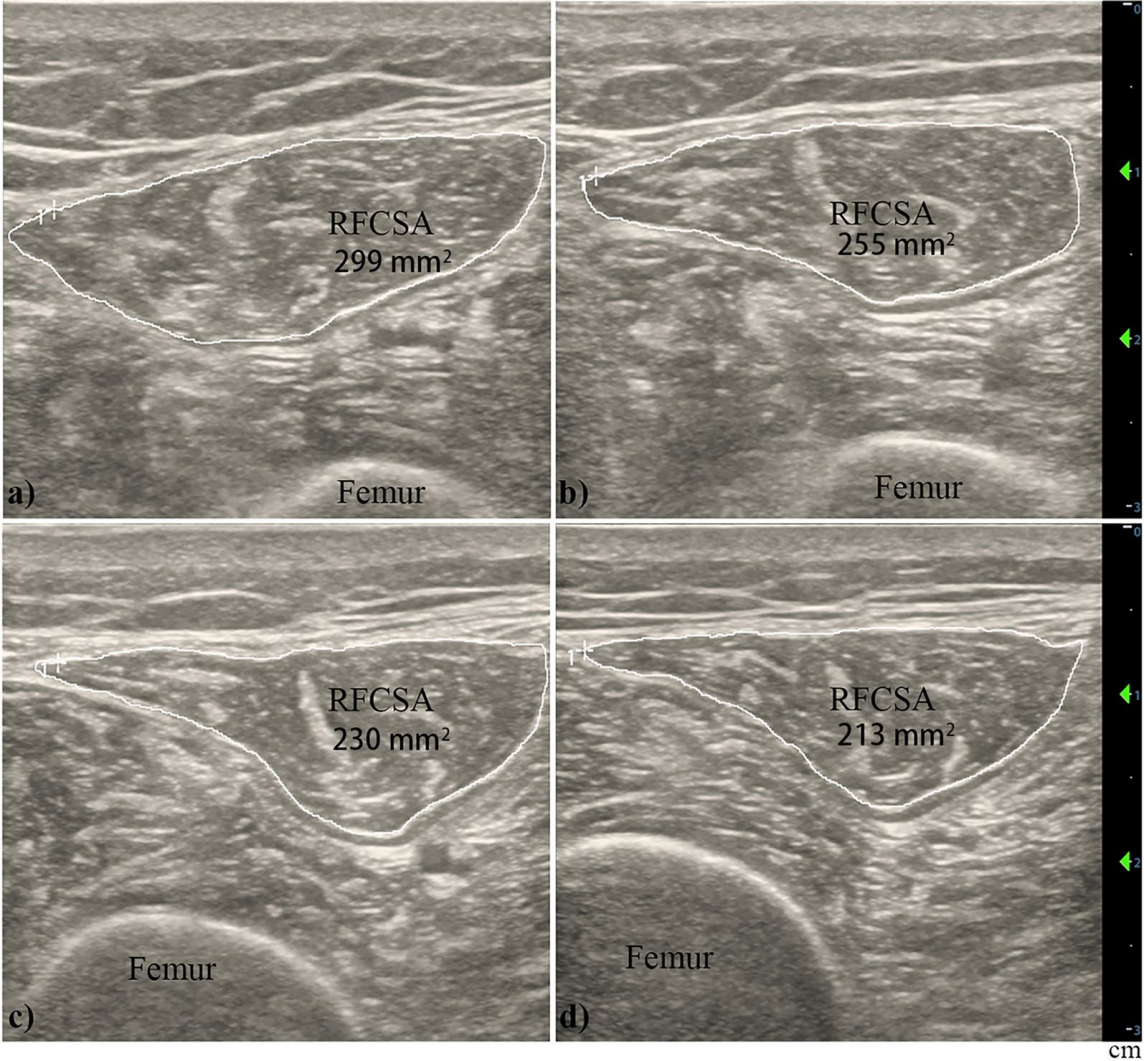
Ultrasound measurements of RFCSA among patients undergoing invasive mechanical ventilation. The RFCSA is outlined by a white border in each image. RFCSA on D1 of ICU admission (a), RFCSA on D3 (b), RFCSA on D5 (c), and RFCSA on D7 (d). The gain and depth settings were consistent across all images. RFCSA, rectus femoris cross-sectional area
